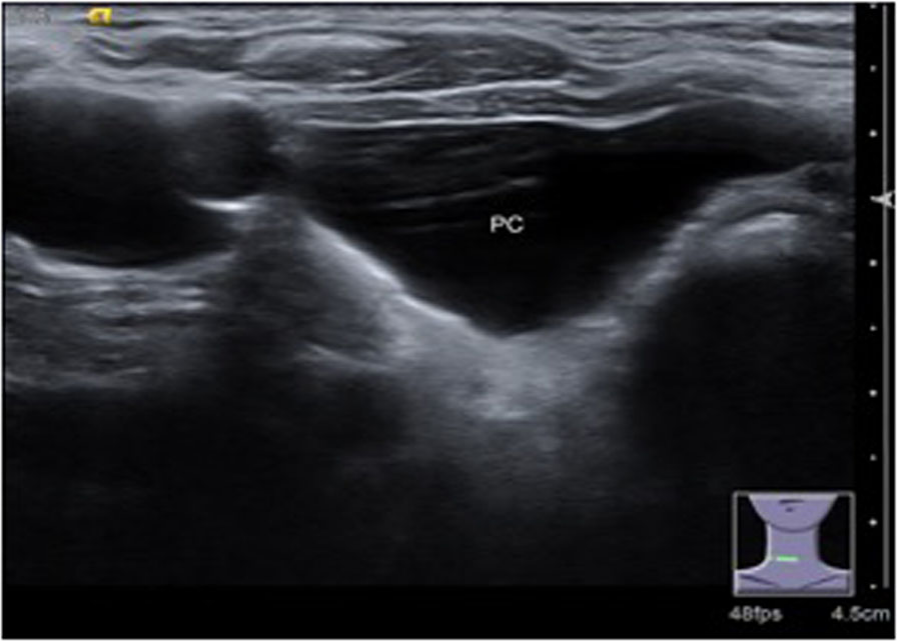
Representative case with PC ultrasonography scans. Cystic lesion (2.5×2×0.8 cm) in right lobe of thyroid gland. PC, parathyroid cyst
